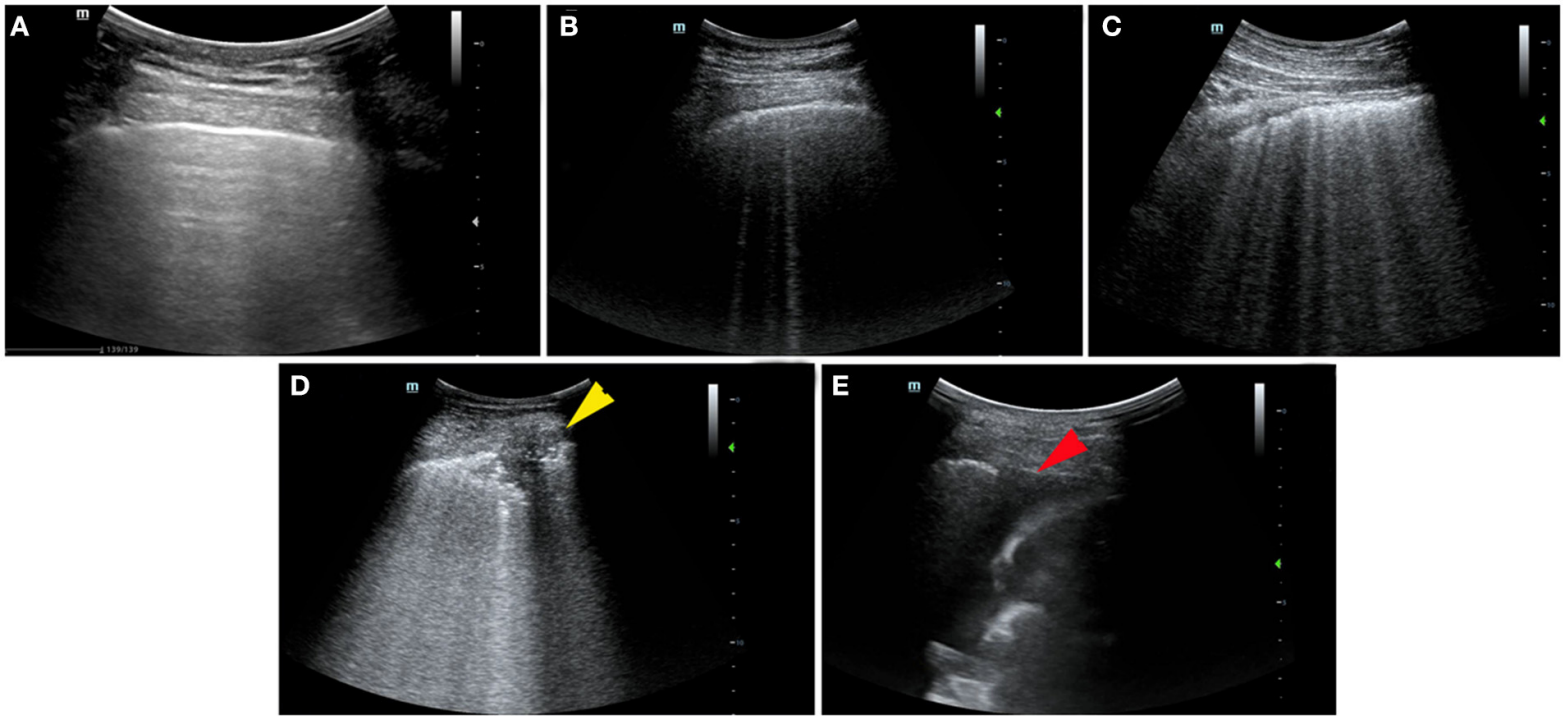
Ultrasonographic features and lung ultrasound (LUS) score in patients with coronavirus disease 2019 (COVID-19). (A) Normal: the presence of A lines beyond the pleural line characterizes mornal pulmonay aeration, LUS score: 0. (B) B1 line: the presence of multiple vertical B lines (comet tails) with well-defined spacing regularly spaced B lines 7 mm apart, LUS score: 1. (C) B2 line: the presence of coalescent B lines <3 mm apart, LUS score: 2. (D) Lung consolidation: the presence of a tissue pattern (yellow arrowhead), LUS score: 3. (E) Pleural effusion at costophrenic angle (red arrowhead).
